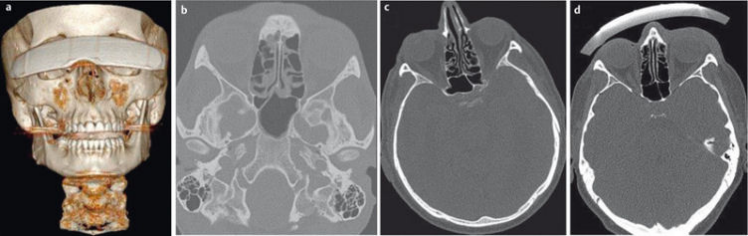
Impact of lens protection devices on image quality. a) 3D-VRT reconstruction. The lens protection device can be worn like glasses. b) Standard CT protocol of the midface without lens protection. c) Low-dose CT protocol without lens protection. Discrete increase of image noise. d) Low-dose CT protocol with lens protection. There is no significant decrease of image quality. Lens exposure is reduced by 58%.
